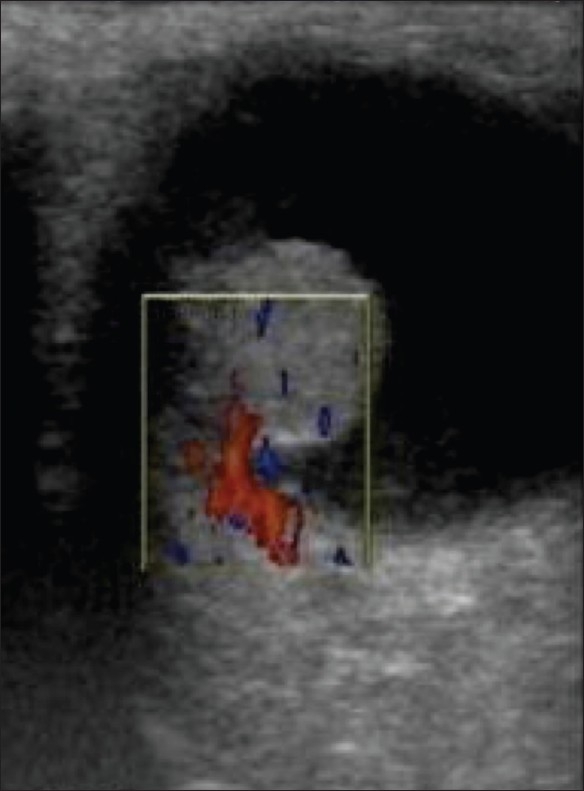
Choroidal melanoma. Color Doppler reveals vascularity in a malignant choroidal melanoma
